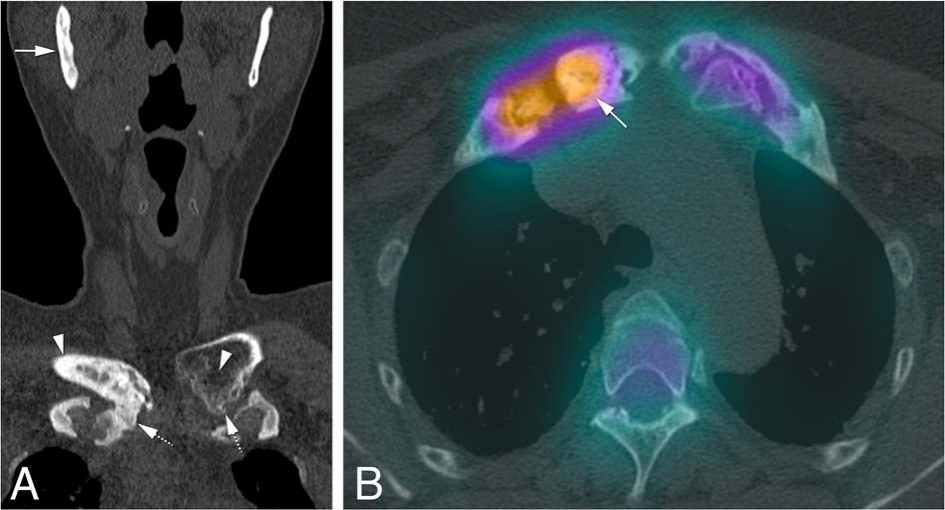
(A) Subtle CNO features at the level of the medial end of the left clavicle in the form of endomedullar bony lucencies (arrowhead), and subtle calcification of the costoclavicular ligament (arrow), while a more advanced stage of the disease can be seen in the contralateral medial end of the right clavicle in the form of sclerosis, hyperostosis and bone erosions (arrowhead), as well as partial ankylosis of the right costoclavicular joint (arrow). Sclerosis and hyperostosis is also seen in the right mandible (arrow). (B) Increased radiopharmacon uptake on SPECT-CT of the right clavicle in the presence of hyperostosis reflecting increased bone formation as also seen in (A), in contrast with the absence of increased radiopharmacon uptake on the contralateral side, which demonstrated only mild abnormalities at the left side.
